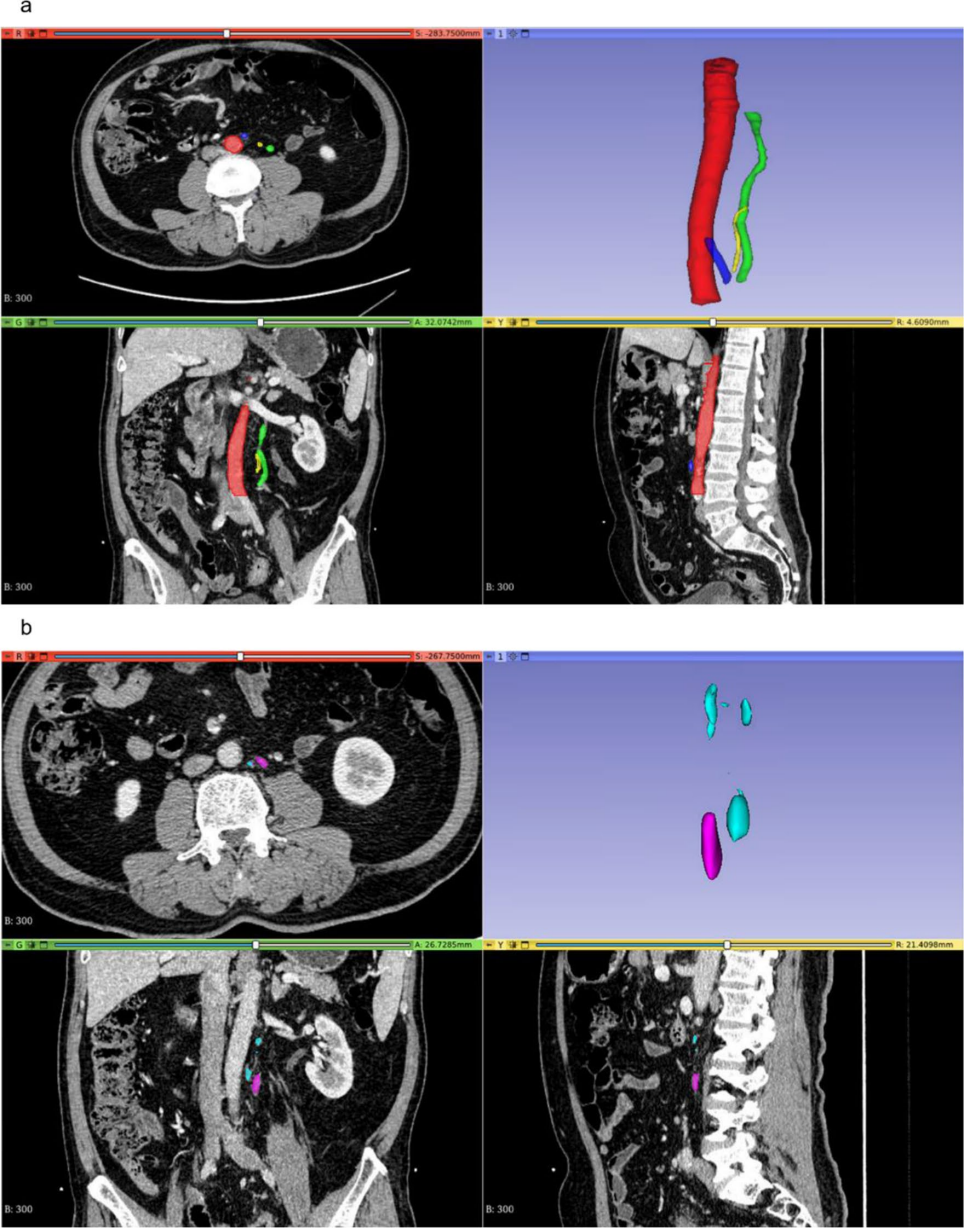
The annotation of No. 253 LN region on CT images (a). The four views represent axial (top left), coronal (bottom left), sagittal (bottom right), and 3D (top right) images. The red area represents the aorta, the green area represents IMV, the blue area represents IMA, and the yellow area represents LCA. The upper boundary for annotating No. 253 LN was the starting point of IMA, with the right boundary being the left edge of the aorta, the left boundary being the right edge of IMV, and the lower boundary extending from IMA to the intersection of LCA and IMV. The annotation of all the lymph nodes in No. 253 LN region (b). The purple area represents the largest lymph node and the light blue area represents other lymph nodes in No. 253 LN region. LN = lymph node; IMV = inferior mesenteric vein; IMA = inferior mesenteric artery; LCA = left colic artery
